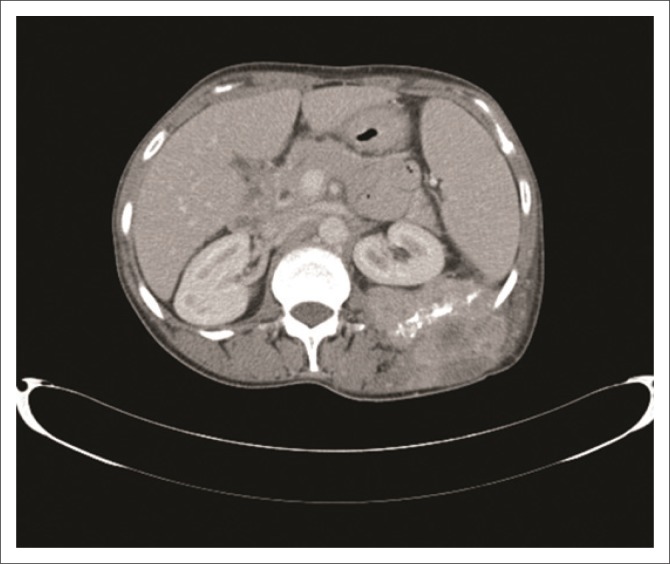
Axial post contrast computed tomography scan image at the level of the 12th rib demonstrating the destruction of the left rib with surrounding soft tissue mass.
